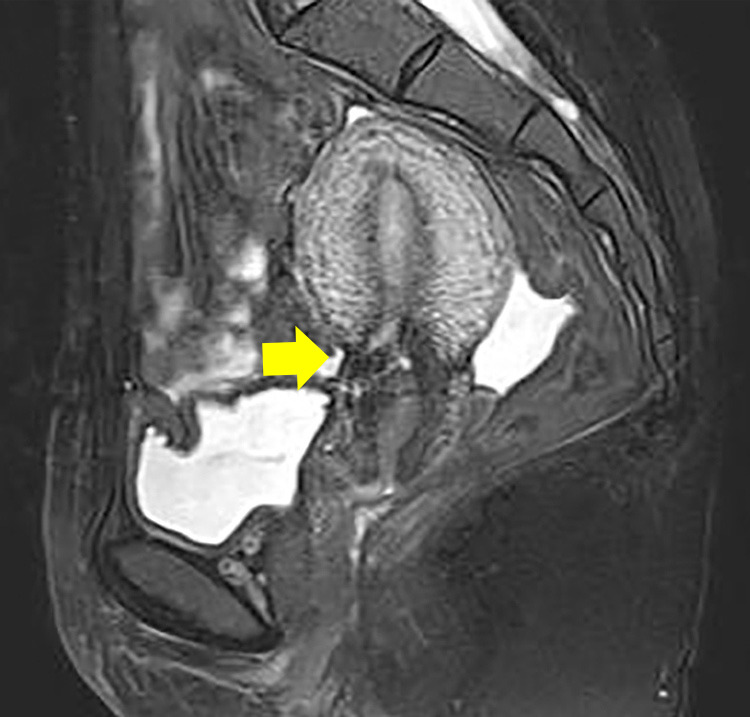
Sagittal simple MRI T2-weighted image: cesarean scar disorder; residual myometrial thickness (RMT) area in CSDi (arrows).
